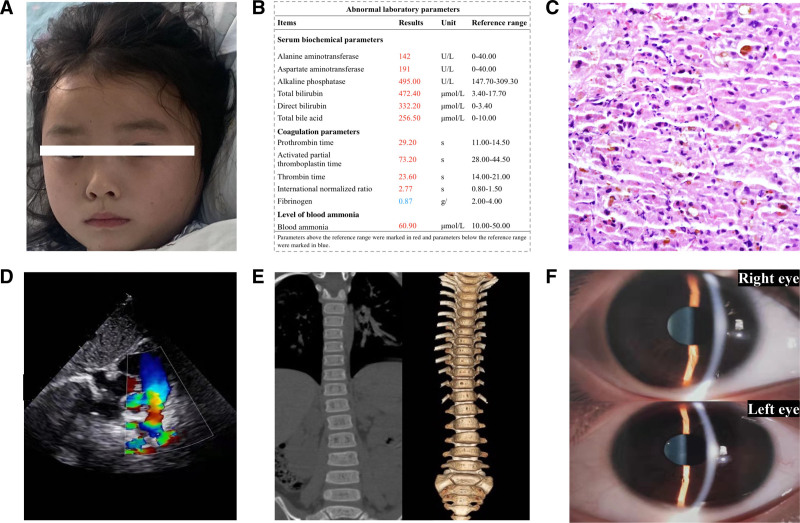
The result of clinical evaluation in the patient. (A) Characteristic facial features were not observed. (B) Abnormal laboratory parameters were detected. (C) Cholestasis was indicated by liver biopsy. (D) Echocardiography revealed pulmonary stenosis. (E) The CT scan and 3D reconstruction of spine showed no obvious abnormalities. (F) Posterior embryotoxon was not found through slit-lamp examination of eyes. CT = computed tomography.
